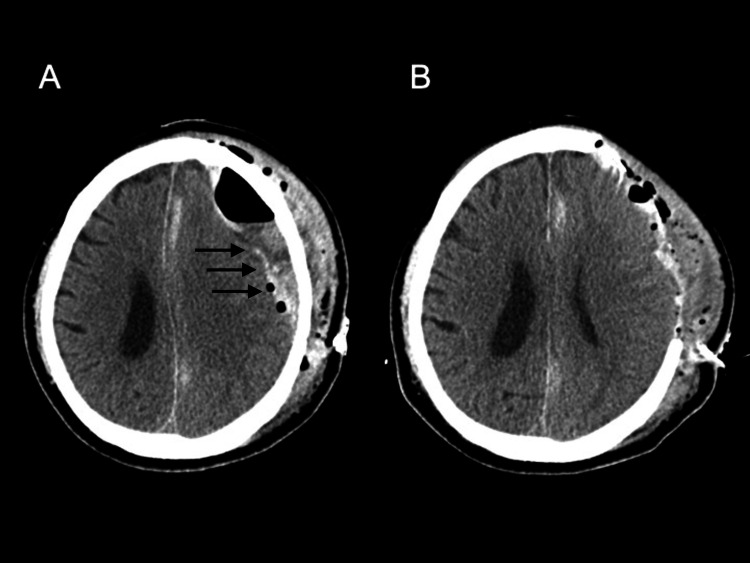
Head CT following hematoma evacuation. (A) Rebleeding (solid arrows) observed after the first craniotomy for hematoma evacuation. (B) Second craniotomy with external decompression performed due to recurrent bleeding.
